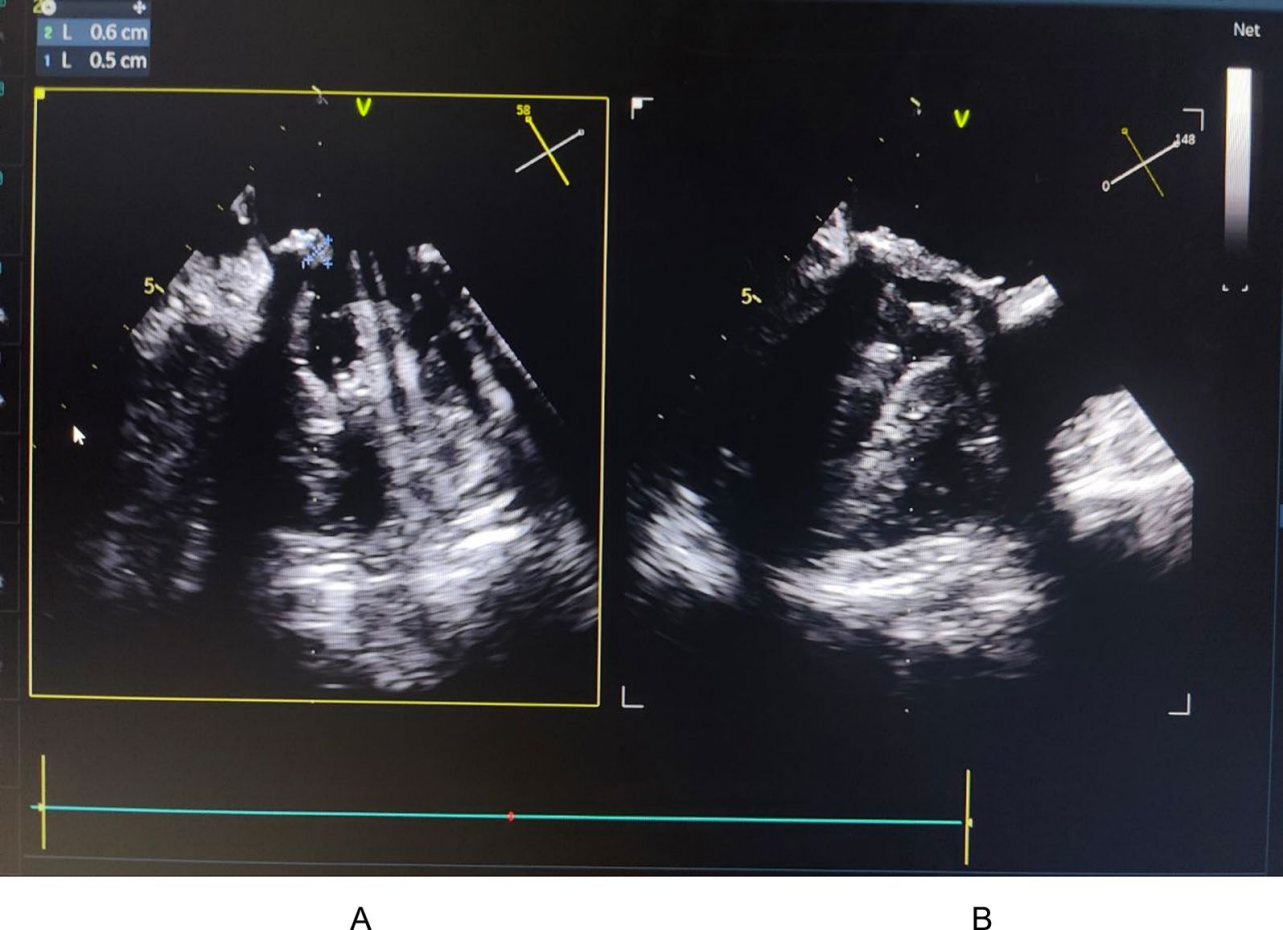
Transesophageal echocardiography images of the mitral prosthesis in mid-esophageal views at 148° (A) and 58° (B), showing a reduction in vegetation size to 5 mm by 6 mm, with resolution of posterior leaflet obstruction and a decrease in the mean transprosthetic gradient to 10 mmHg
